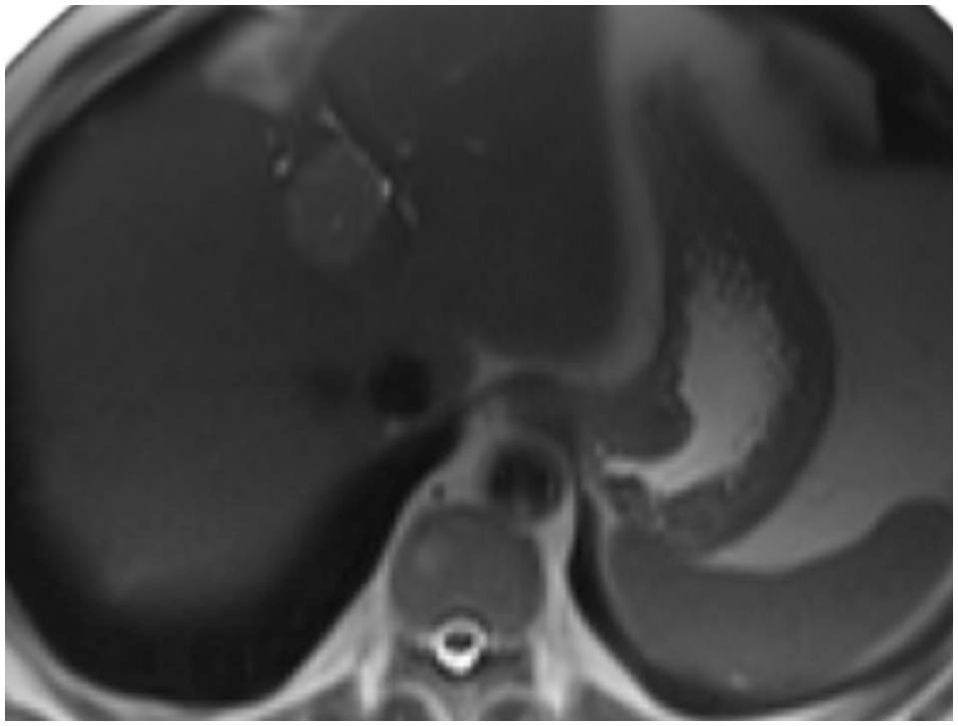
Axial MRI slice showing a well‑circumscribed submucosal mass on the lesser curvature.
